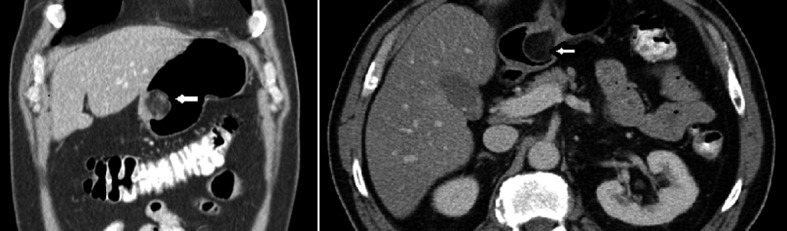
Axial and sagittal view of CT scan abdomen and pelvis showing a polypoid mass (white arrows) arising from gastric antrum.
